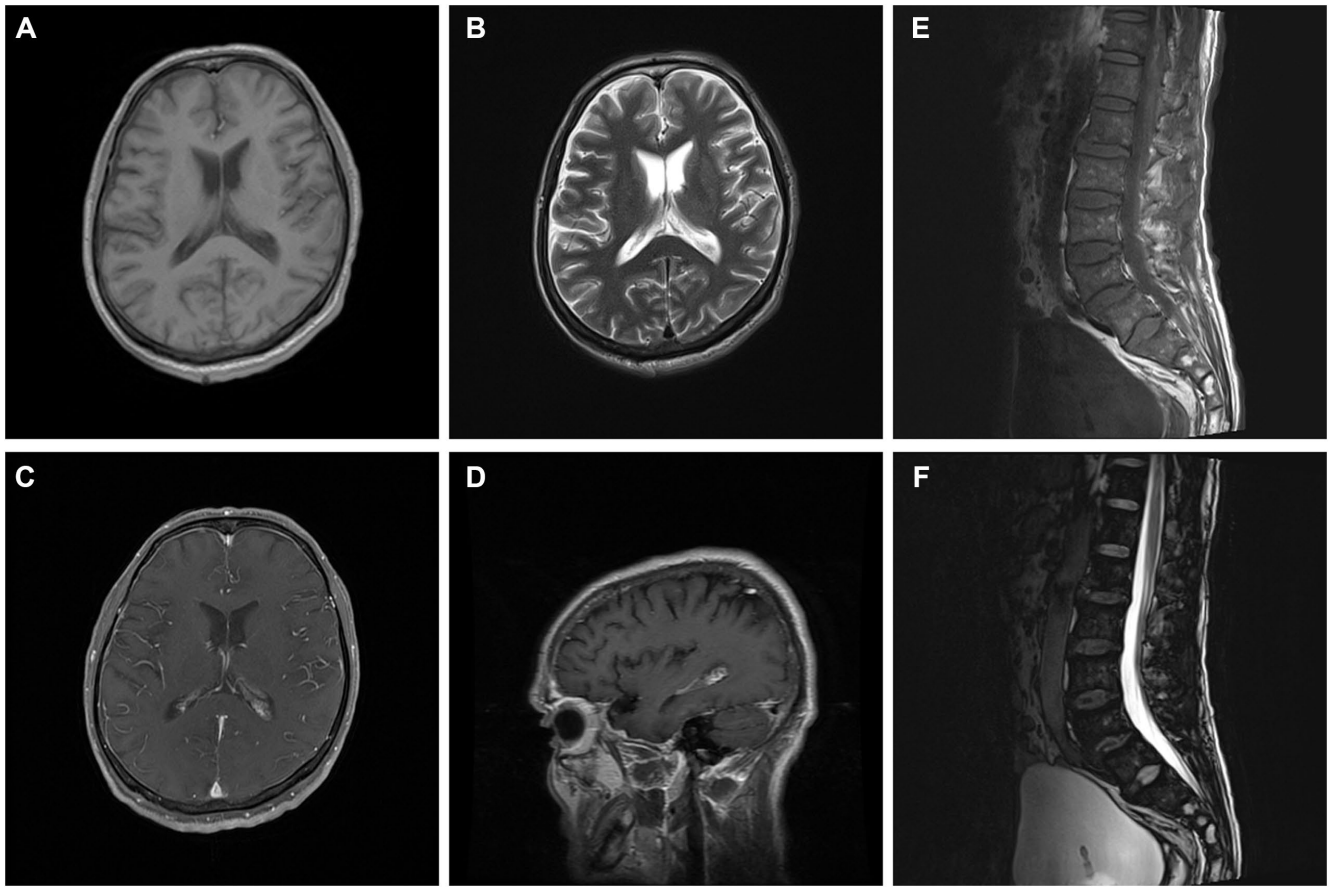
Brain and lumbar MRI. Brain images showed meningeal enhancement and enlargement of arachnoid villi (A–D). Lumbar images showed spinal disc herniation and lumbar intervertebral space edema (E,F).
